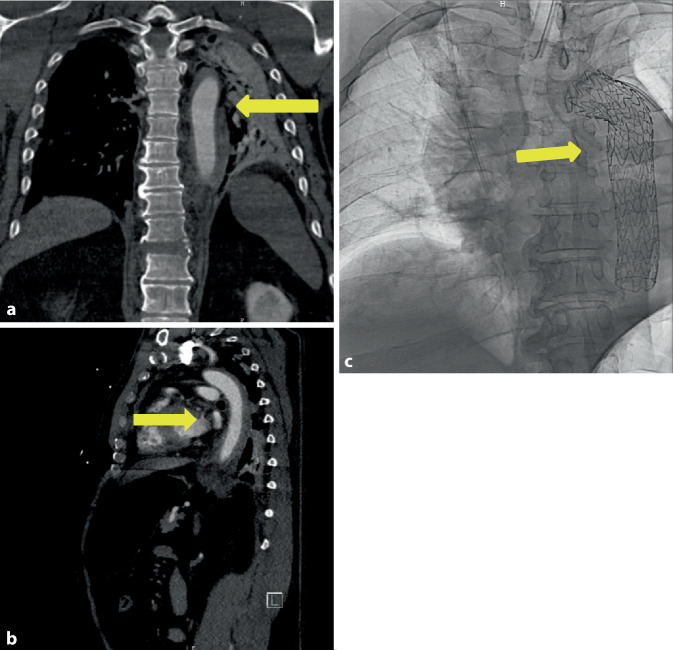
Fall 6: Traumatische Dissektion/Hämatom der Aorta thoracica nach Verkehrsunfall. a CT-Angiographie mit koronarer Darstellung des Aortenwandhämatoms (*gelber Pfeil*), b CT-Angiographie mit sagittaler Darstellung des Aortenwandhämatoms (*gelber Pfeil*), c Nativröntgenbild des Aortenstents (*gelber Pfeil*)
